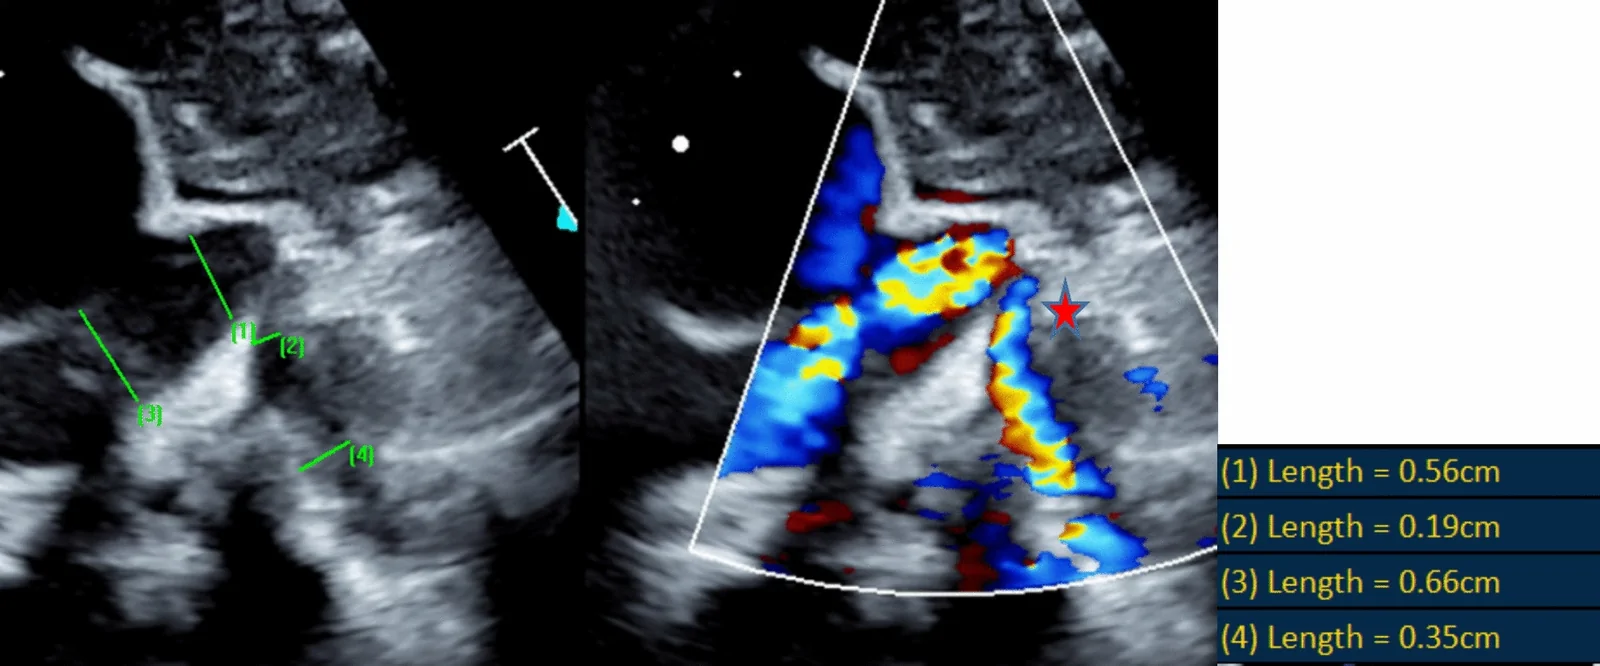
2D vs color Doppler echo images used to assess both categorical pulmonary artery stenosis (star) and quantitative pulmonary artery measurements six months after PDA stenting, (1&2) represent proximal branch pulmonary artery measurements, while (3&4) represent distal measurements used to calculate McGoon ratio on echo
